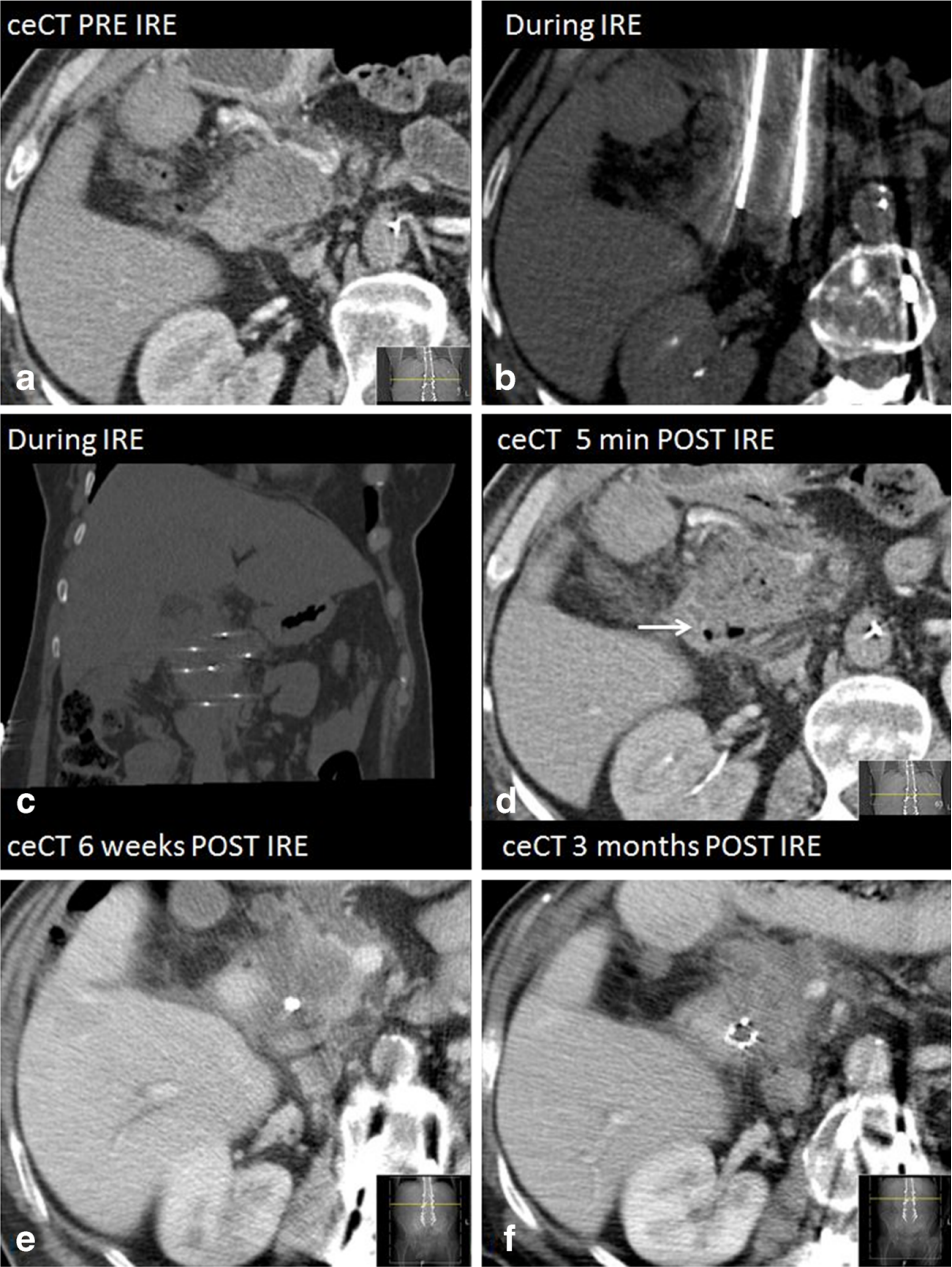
Imaging findings during follow-up on ceCT (a) Isoattenuating tumour on ceCT pre-IRE (b) CT-guided placement of electrodes around the outer border of the tumour (c) Confirmation of correct electrode configuration according to the treatment plan with a nonenhanced CT scan (d) Hypoattenuating IRE ablation zone with intralesional gas pockets immediately after IRE (e) Hypoattenuating IRE ablation zone at 6 weeks of follow-up (f) Hypoattenuating IRE ablation zone at 3 months of follow-up
